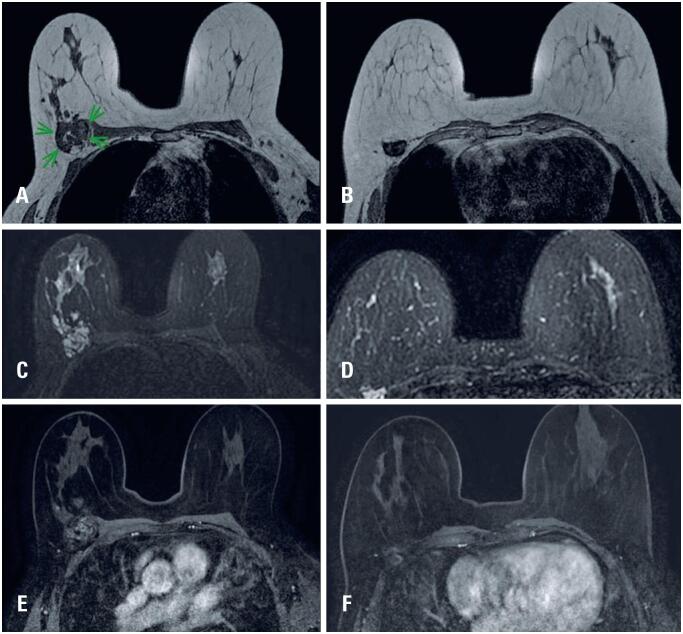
T1-weighted breast MRI showing two irregular masses in the posterior third of the upper outer (A) and lower outer (B) quadrants of the right breast. T2-weighted breast MRI showing both lesions (C, - upper outer quadrant, D, -lower outer quadrant) with high signal intensity. Post-contrast subtraction images demonstrate heterogeneous and persistent enhancement of the lesions (E, - upper outer quadrant; F, - lower outer quadrant), without evident enhancement the pectoralis major muscle
